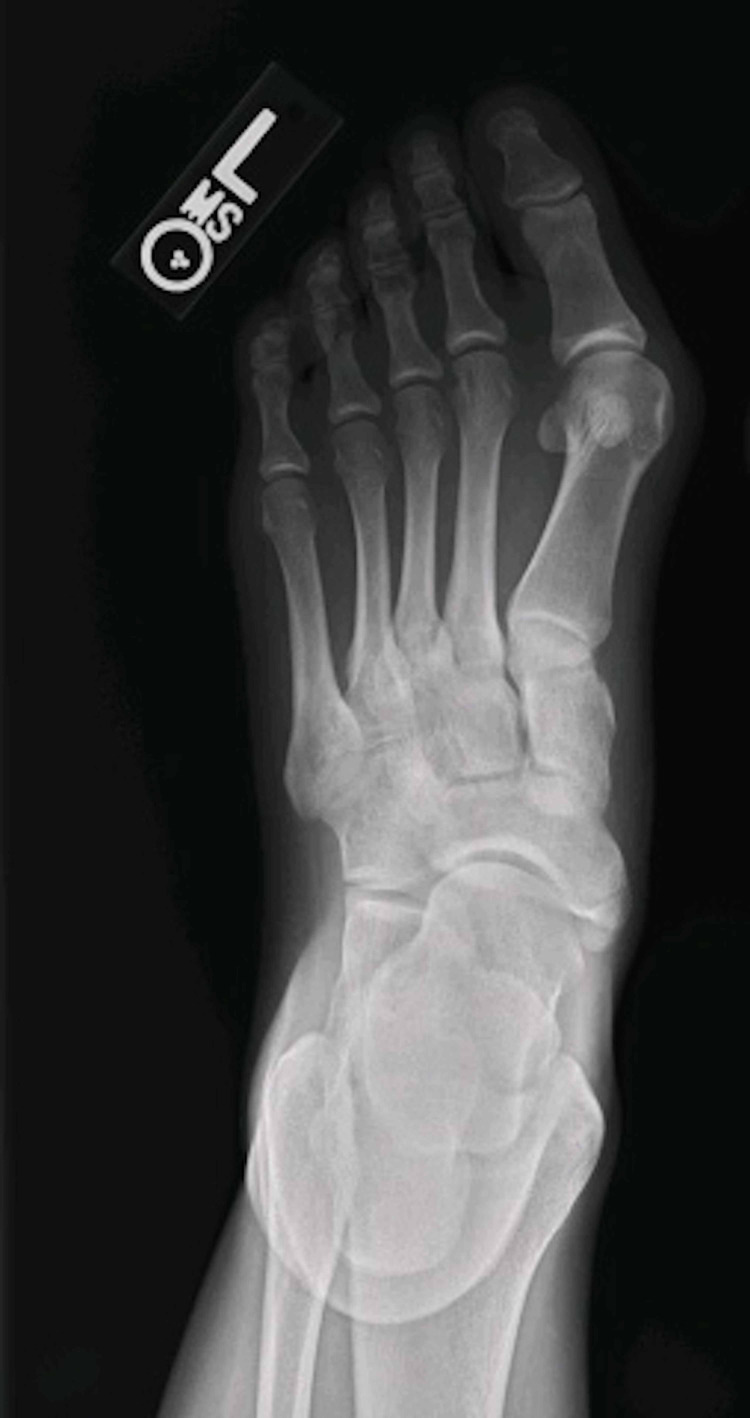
Anteroposterior radiograph demonstrating a preoperative foot with hallux valgus deformity.
